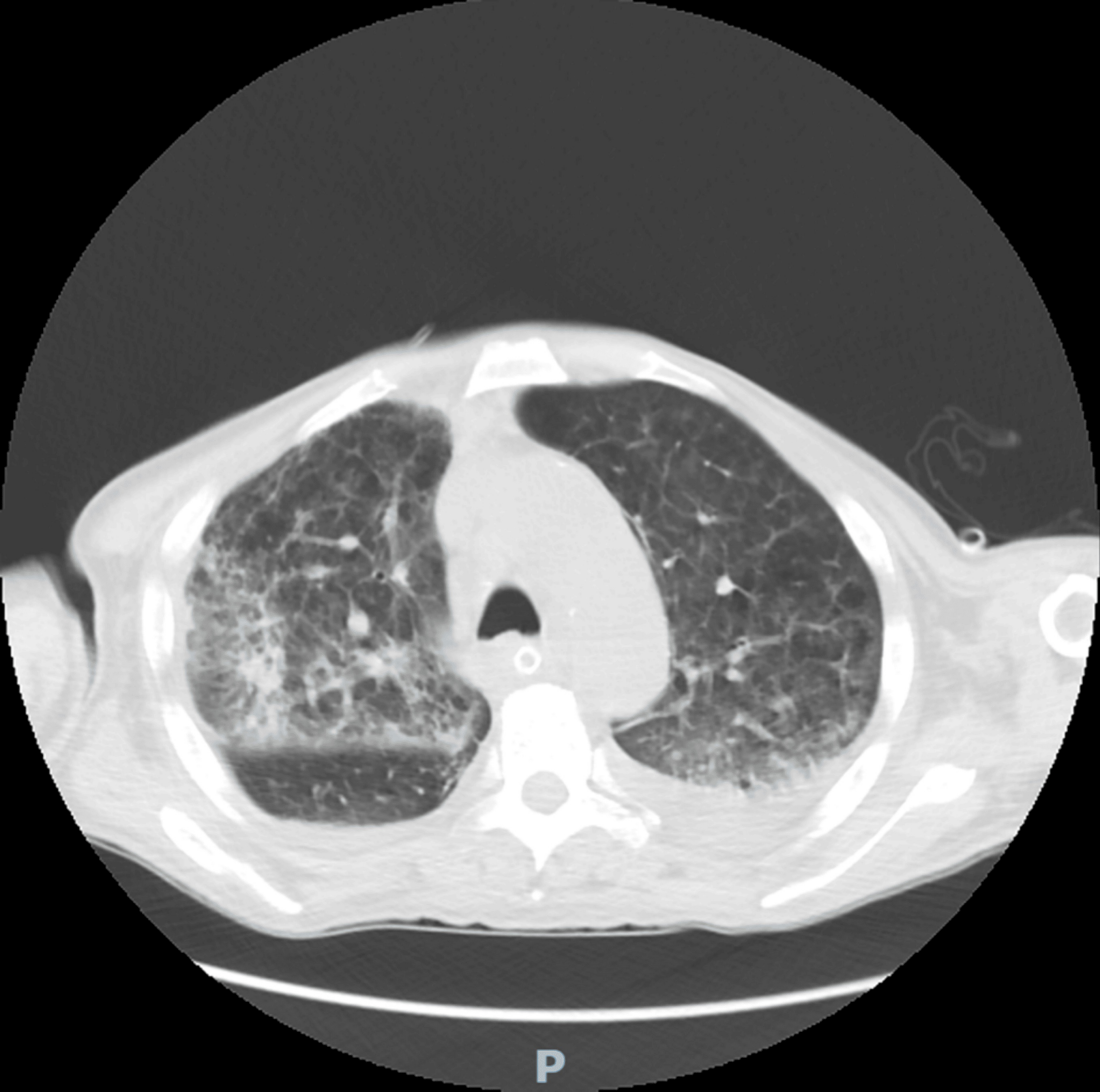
The chest CT scan performed one month after admission described bilateral irregular consolidations with an air bronchogram, primarily of inflammatory etiology, with signs of perilesional bronchiolitis, and then bilateral reticular changes in the interstitium and ground-glass opacity (GGO) zones.
